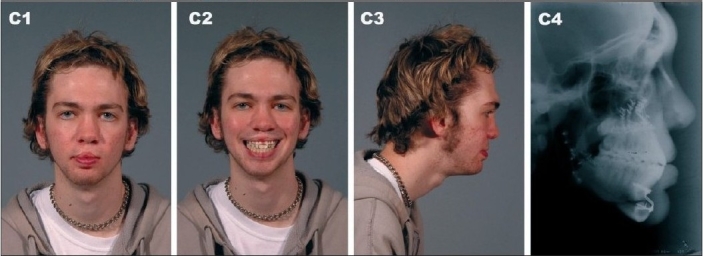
(C) 18 years of age showing long term outcome after 3-piece Lefort I osteotomy with maxillary advancement, bilateral mandibular ascending ramus sagittal split osteotomy and genioplasty. (C1) Frontal view (C2) Frontal smiling (C3) Right lateral view (C4) Postoperative lateral cephalometric roentgenogram
